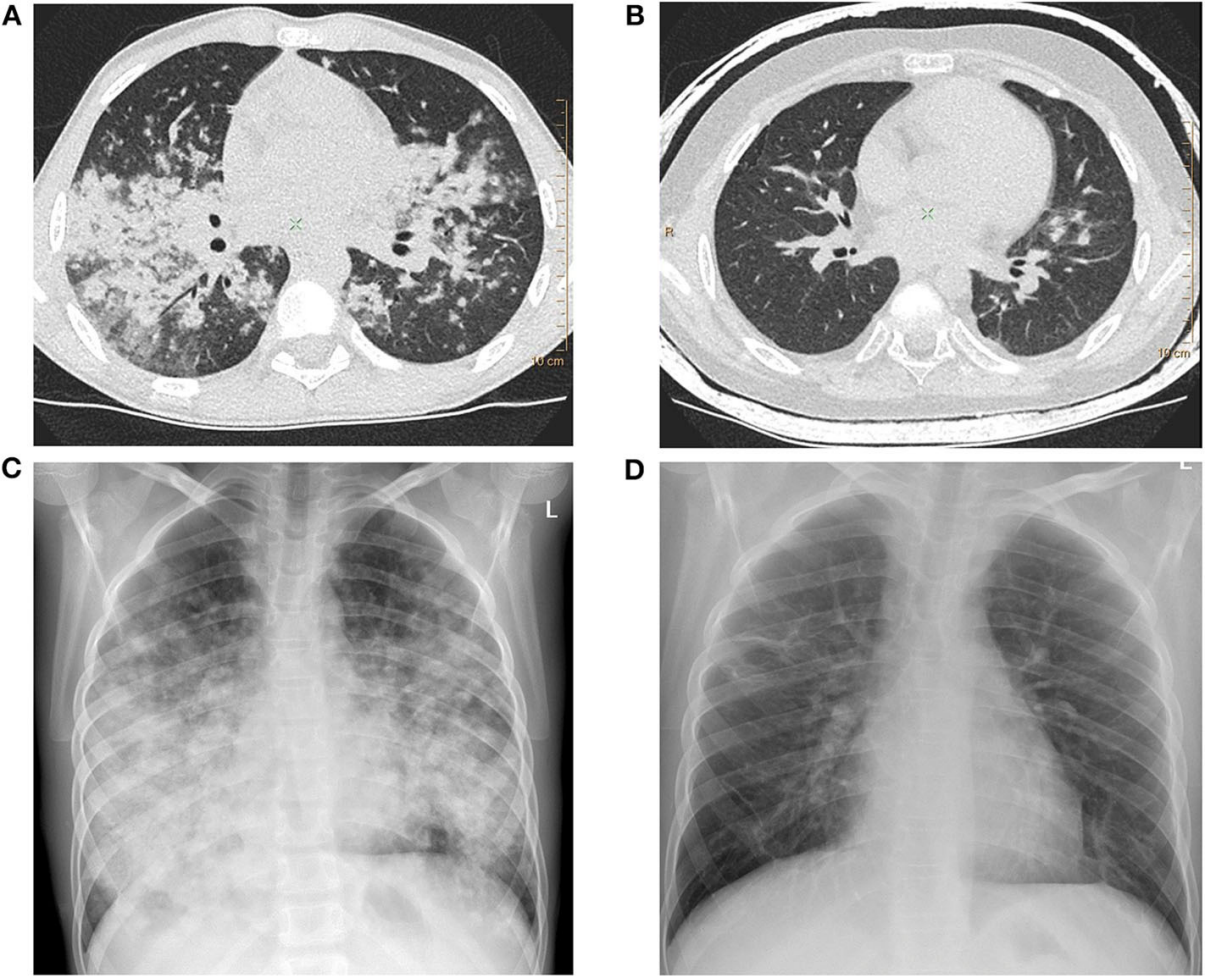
(A) Lung high-resolution computed tomography (HRCT): multiple, poorly defined nodules merging in larger areas of ground-glass opacities and consolidations. (B) Lung HRCT: complete regression of pulmonary changes; residual irregular linear opacities are visible. (C) Chest X-ray: diffuse poorly defined nodules creating larger areas of lung consolidations with adjacent areas of ground-glass opacities. Middle and lower zone predominance is clearly marked. (D) Chest X-ray: complete resolution of pulmonary opacities with residual irregular linear thickenings.
